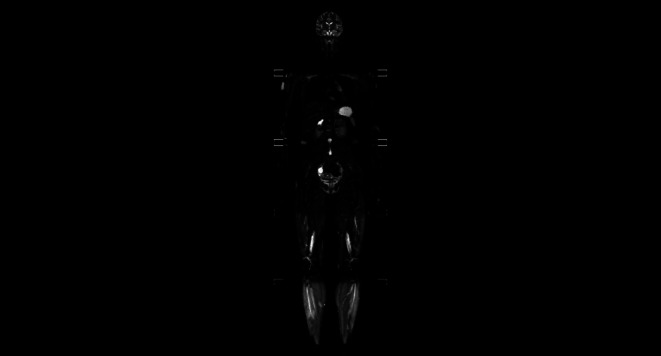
Whole‐body MRI performed 5 years after the onset of the lesion, highlighting the presence of extensive inflammation in the lower limbs.
